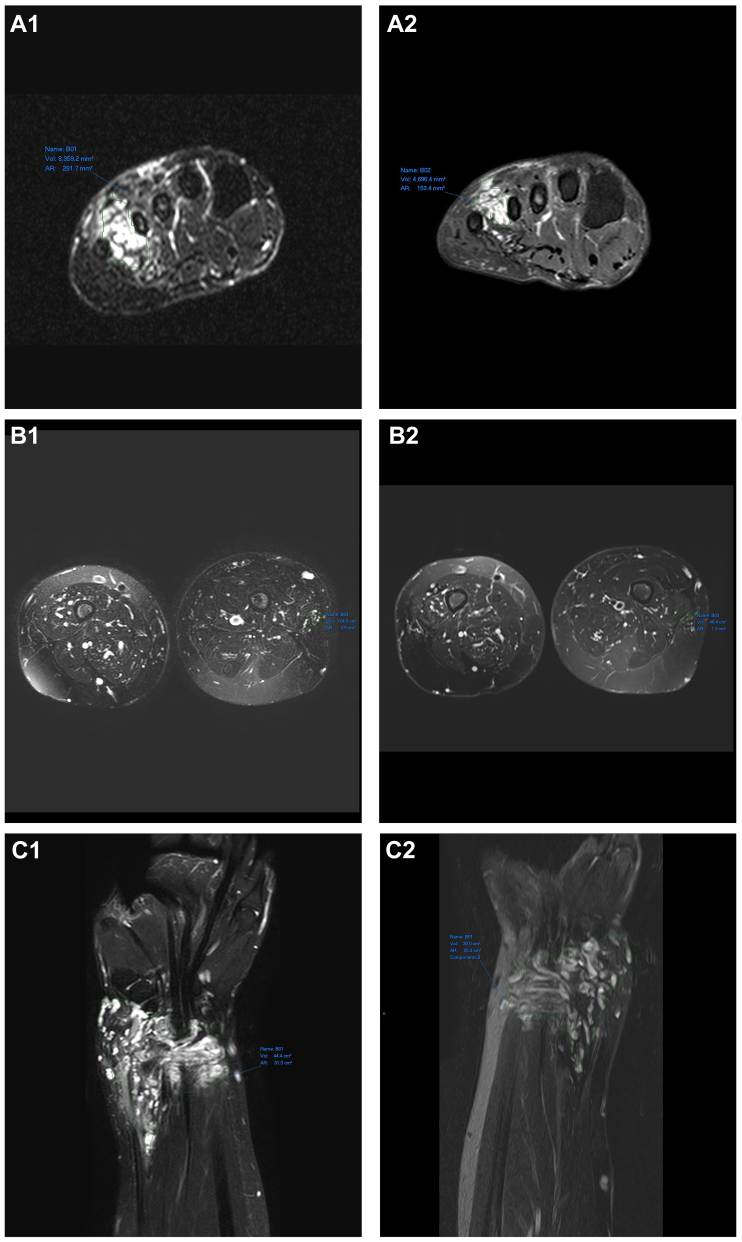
Magnetic resonance imaging (MRI) before treatment (A1-C1) and after treatment (A2-C2) with sirolimus. The lesion volume decreased in these three patients.
